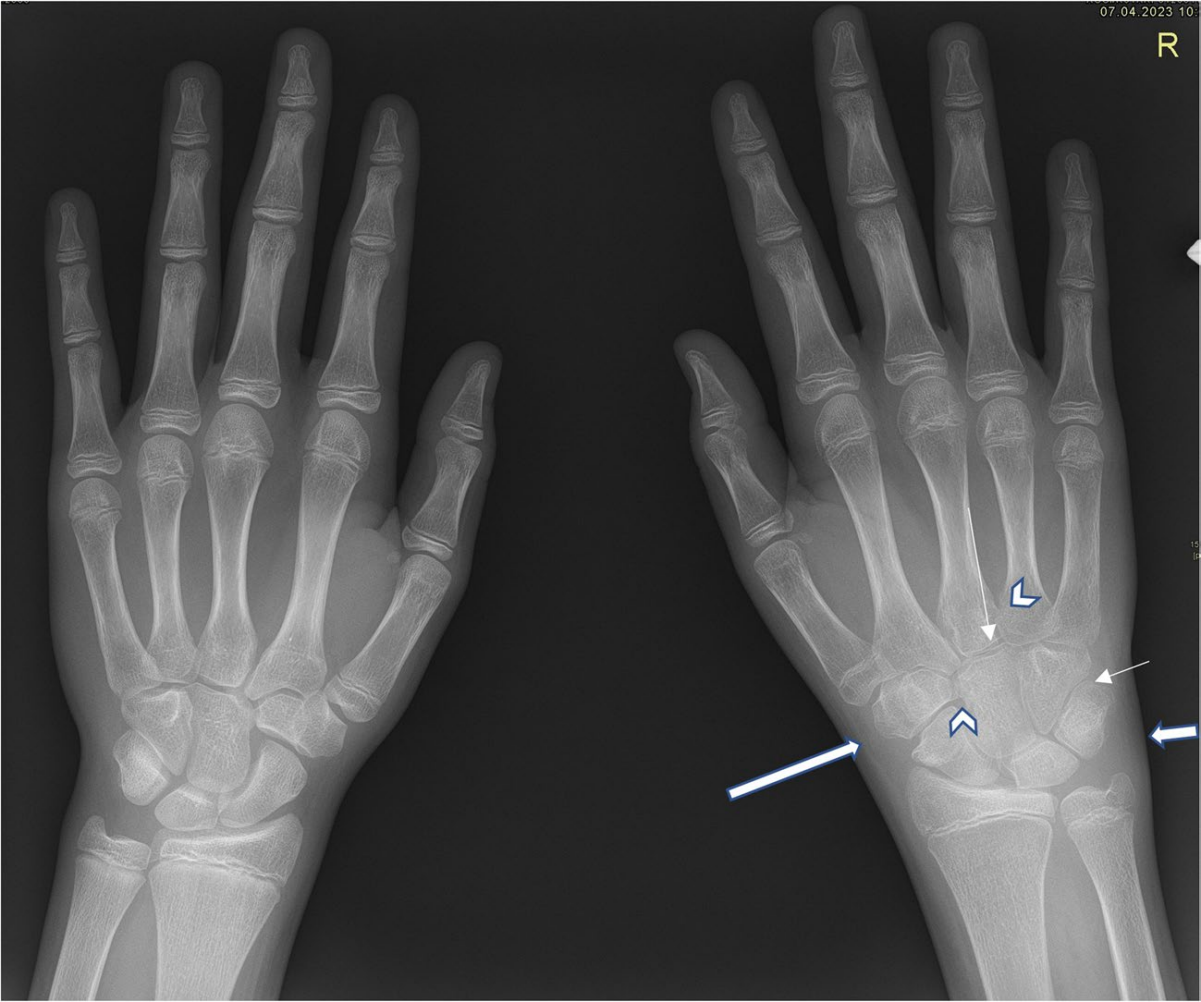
Anteroposterior radiograph of both hands in a 13.5-year-old boy with enthesitis-related (HLA B27 positive) juvenile idiopathic arthritis: general mild osteoporosis of the right hand (*long arrow*), soft tissue edema around the carpal bones on the right (*short arrow*), narrowed carpometacarpal joint spaces on the right (*long thin arrow*), discrepancy of the size of the carpal bones due to accelerated growth on the right (*short thin arrow*), suspicious erosions at the base of the second to fourth metacarpals and at os trapezoideum and os capitatum (*arrowheads*). Normal structure and morphology of the bones of the left hand
